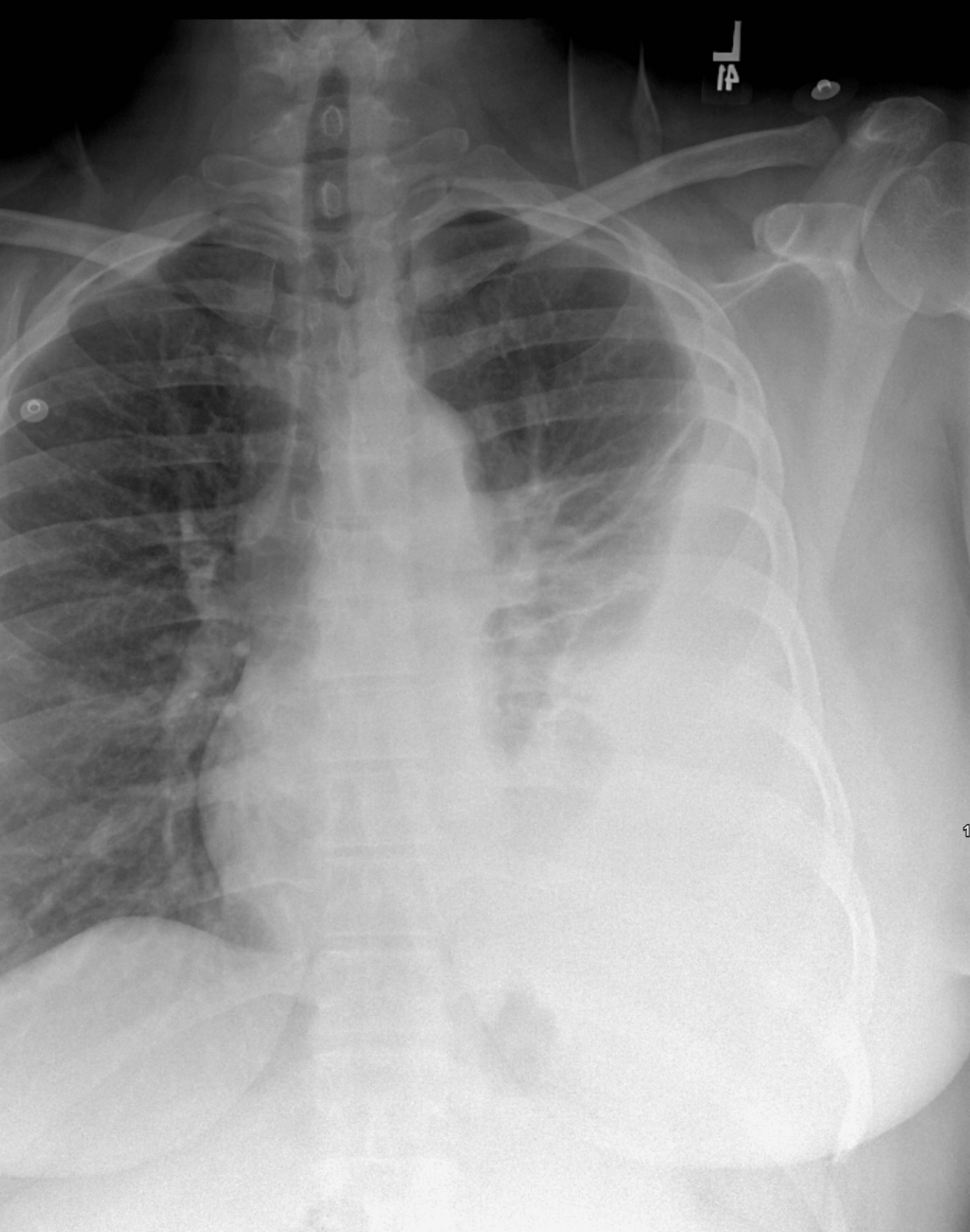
Chest X-ray showing left lower lobe pleural effusion with subjacent atelectasis.
